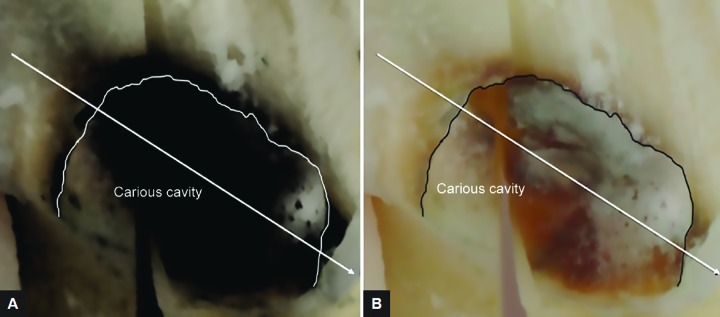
The changes in color and contrast between (A) and (B) of a horizontal cut of a carious cavity cleaned of its softened dentin show an area in black behind the tracing of the boundary of the lesion. It is this area that leads to radiographic overestimation of the depth of the carious lesion
